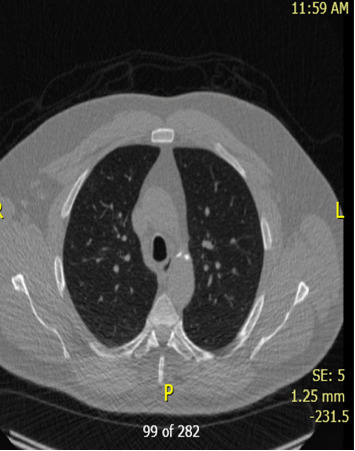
Chest CT findings. CT image of the chest showing volume loss in the right middle lobe, with fissural displacement suggestive of persistent atelectasis. These findings raise concern for possible endobronchial obstruction.
